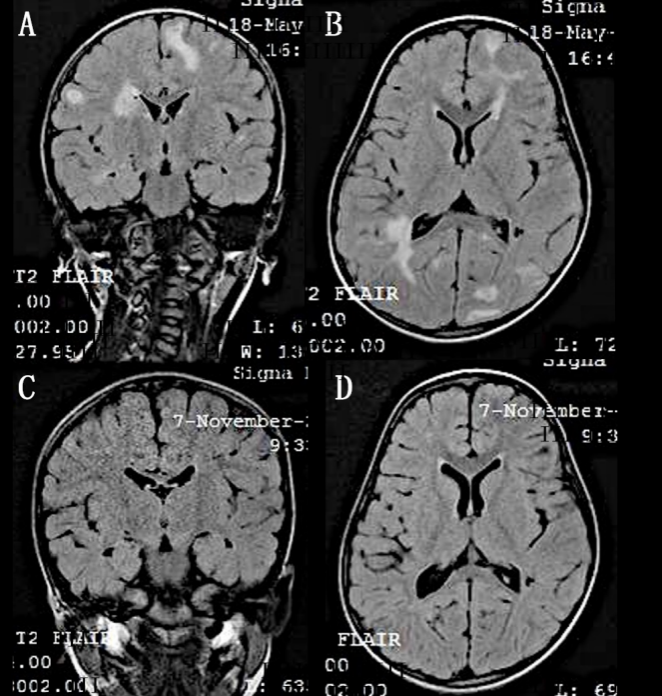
A, B) MRI brain showing enhancing lesions with perilesional edema in the supratentorial and infratentorial brain parenchyma. C, D) These lesions disappeared following treatment.
